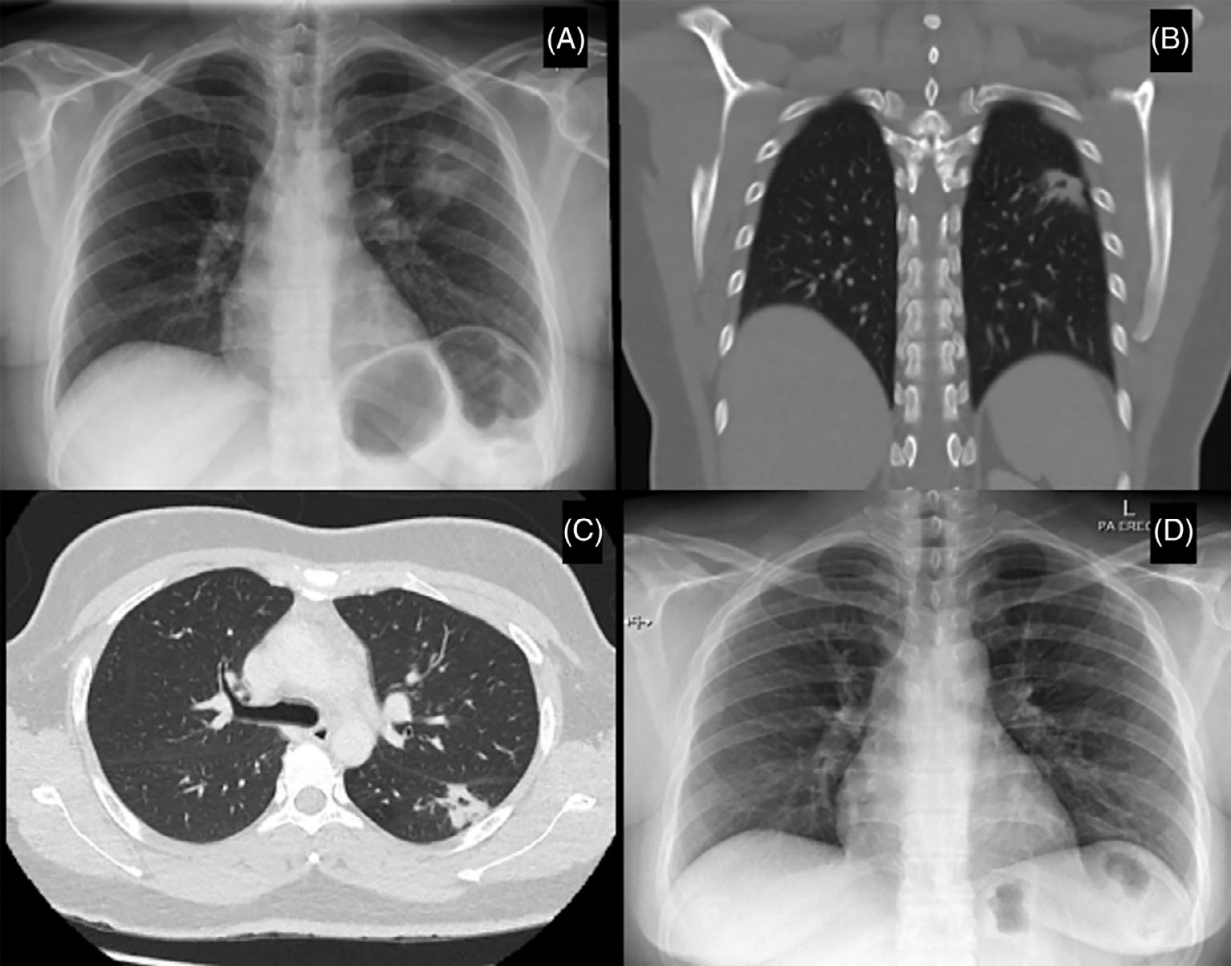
Chest radiograph (A) on initial presentation showed left midzone cavity. Coronal (B) and axial (C) views of the computed tomography thorax revealed spiculated thick‐walled cavitating lesion at the superior segment of the left lower lobe abutting the oblique fissure. Chest radiograph (D) after 6 months of corticosteroids therapy revealed resolution of the left midzone cavity
